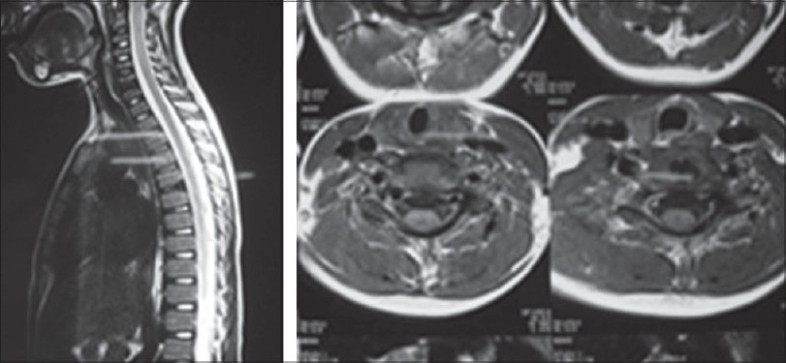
MR images showing hypointense signals at the levels of calcified discs
